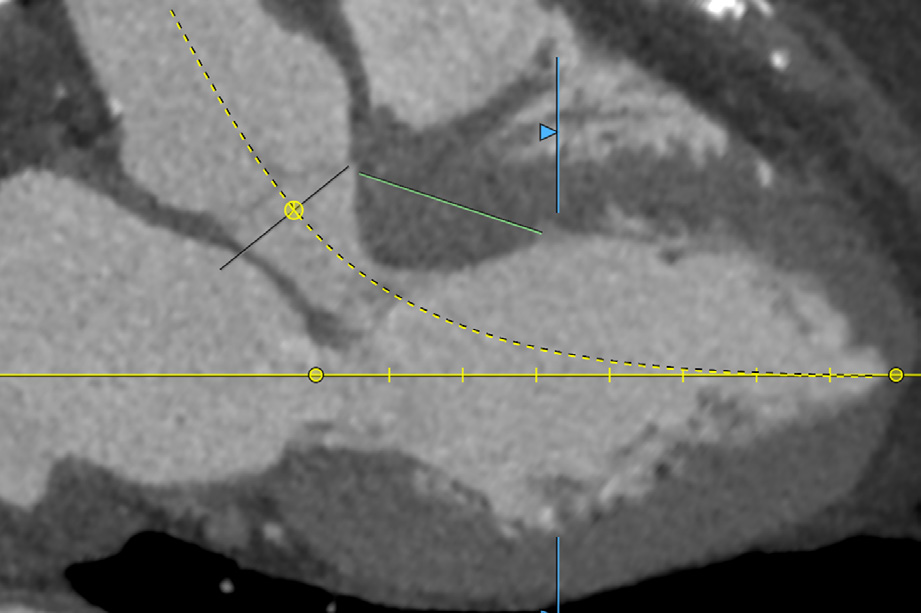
The Septal Scoring Along Midline Endocardium trajectory (green line) is planned on computed tomography to shave the hump and to enter the septum immediately below the aortic valve.
